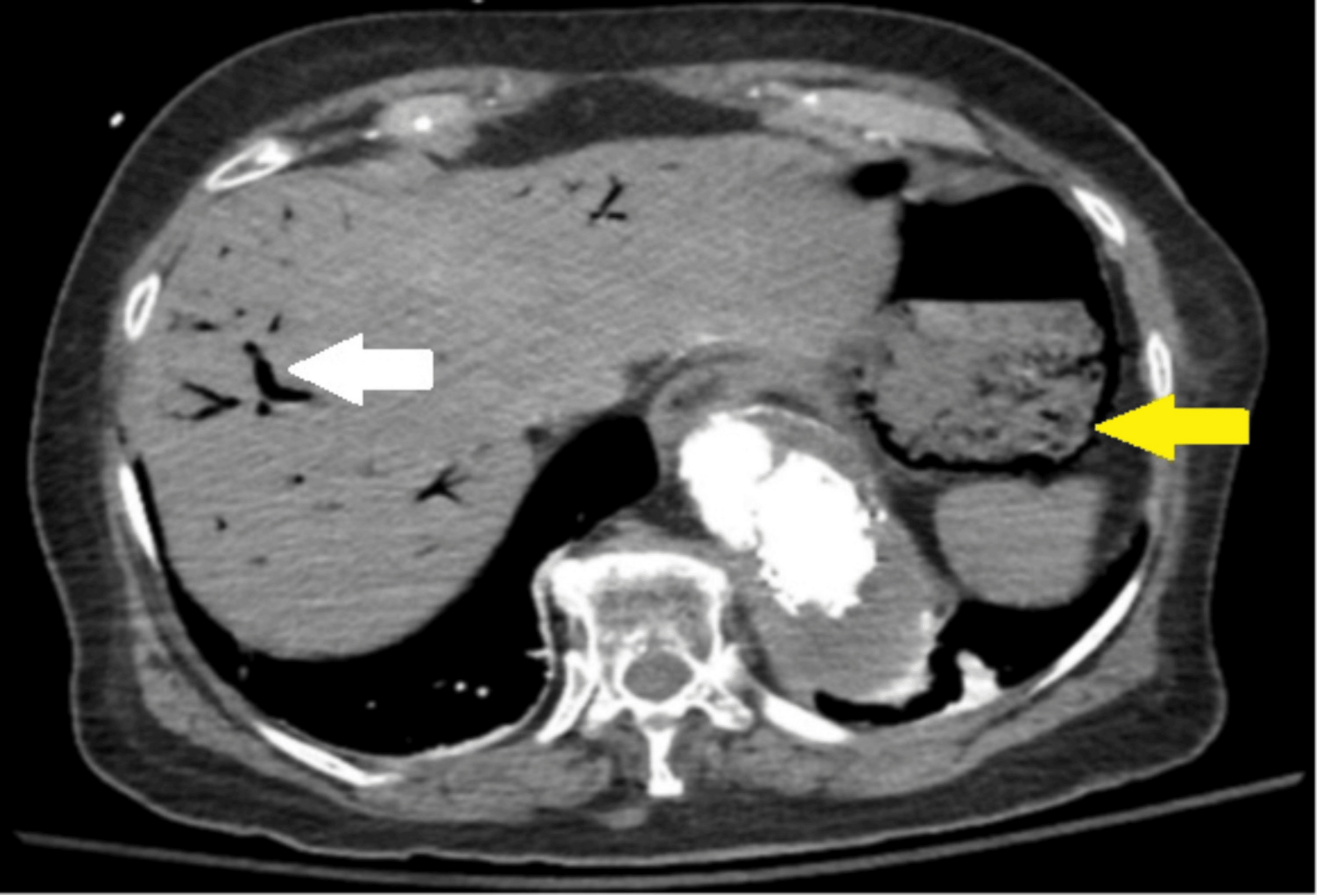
Computed Tomography Angiography (CTA) of the Abdomen and Pelvis CTA demonstrates acute gastric pneumatosis (yellow arrow) and hepatic pneumatosis (white arrow).
